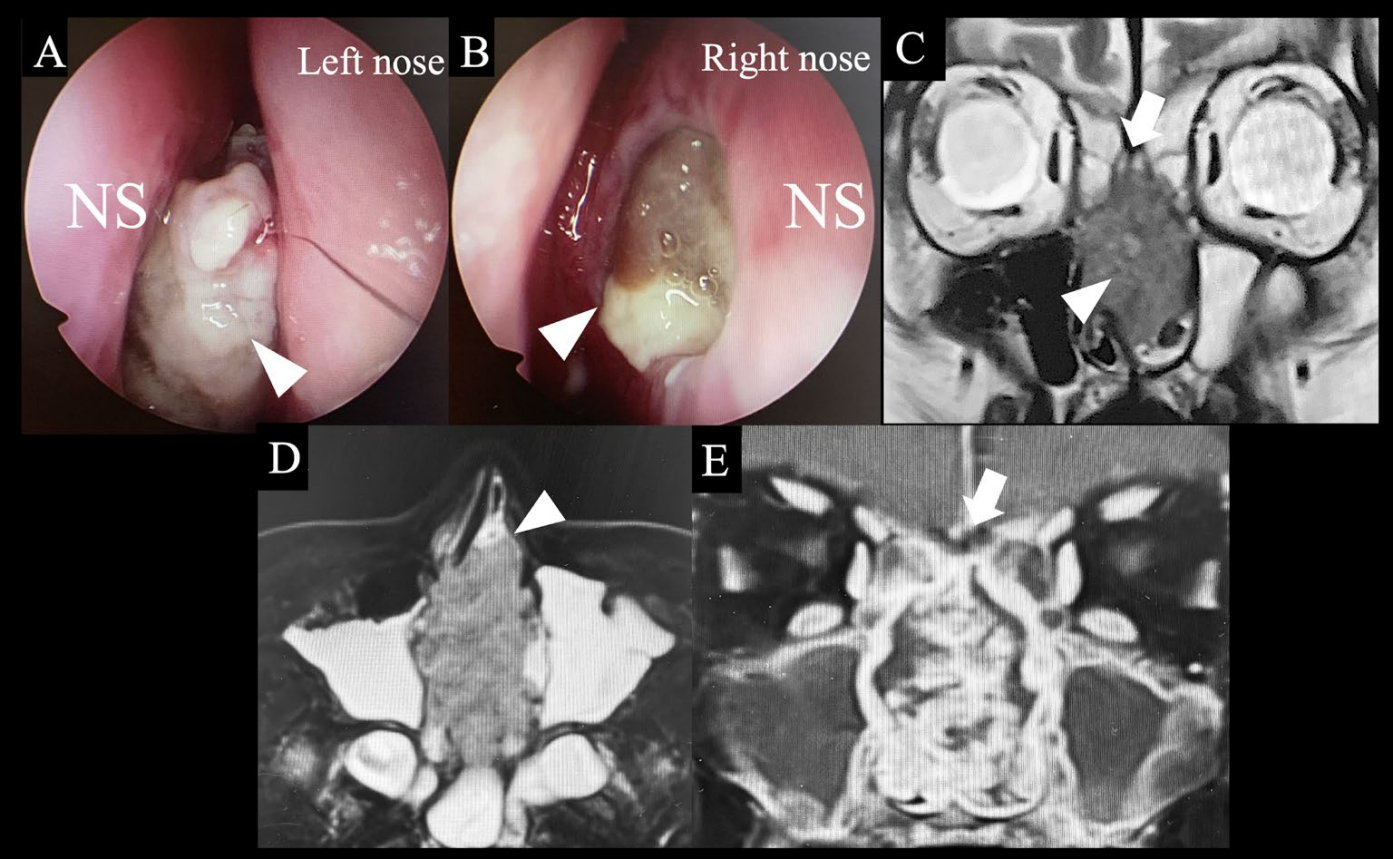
Endoscopic endonasal images before induction chemotherapy. Left (A) and right (B) images. Tumor occupying the nasal cavity, predominantly on the left side is observed (arrowhead). The tumor on the left side is also visible on the right side through perforation of the nasal septum. Coronal (C), axial (D) T2‐weighted and coronal contrast‐enhanced TSE DIXON (E) magnetic resonance image obtained before induction chemotherapy. The tumor is centered on the nasal septum (C arrowhead) and extended anteriorly to the margin of the piriform aperture (proximally only, no mucosal or skin invasion, D arrowhead), cephalad to the cribriform plate (proximally only, no obvious invasion, C and E arrow), lateral to the bilateral middle turbinate and posterior to the front of the anterior wall of sphenoid sinus. NS: Nasal septum.
